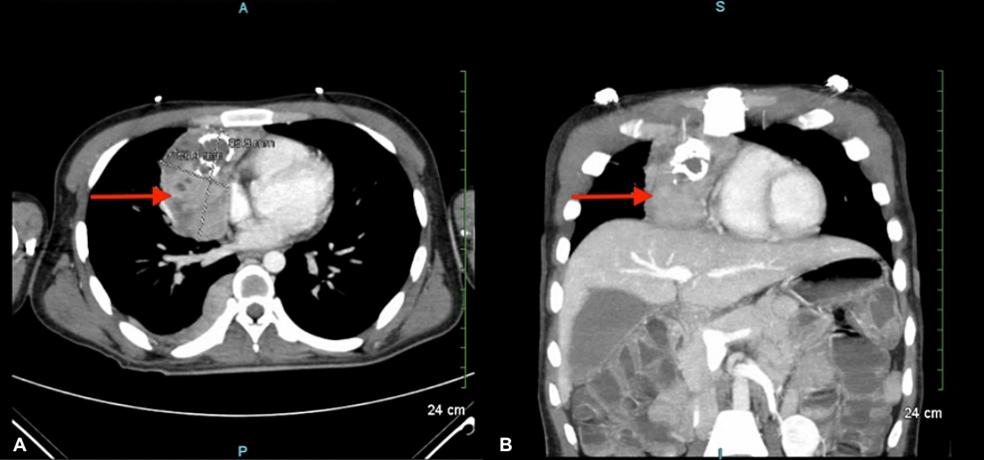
A: Axial section of chest CT with IV contrast showing a large right mediastinal mass and right posterior pleural thickening. B: Coronal section of chest CT with IV contrast showing a large right mediastinal mass with irregular prior to thymectomy and debulking. CT: computed tomography, IV: intravenous
